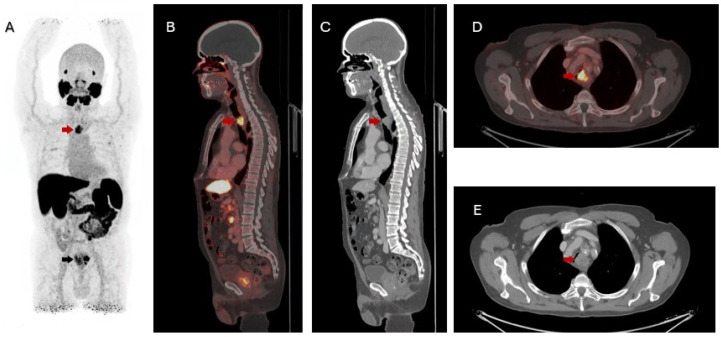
Pretherapeutic ^18^F-PSMA PET/CT of a 75-year-old man with high-risk prostate cancer (biopsy confirmed typical adenocarcinoma, Gleason 9 [4+5], cT3bN0M0 and PSA 26.1 ng/mL) in maximum intensity projection (A) shows intense uptake in the prostate (SUVmax 29, black arrow) and a focal tracheal lesion (SUVmax 9.1, red arrow), corresponding to a miPSMA score of 2 based on PROMISE criteria. Clinically, the patient had progressive respiratory symptoms, including dyspnea and reduced exercise tolerance. Sagittal fused PET/CT (B) and contrast-enhanced CT (C) demonstrate a 2.9 × 2.2 cm left lateral tracheal mass at Th2–3. Axial fused PET/CT (D) and CT (E) reveal near-complete luminal obstruction and possible mediastinal and esophageal involvement. The findings raised suspicion for a primary lung malignancy versus metastasis; due to the lack of nodal or osseous metastases, a non-prostatic pathology was suspected, and a biopsy was recommended.
